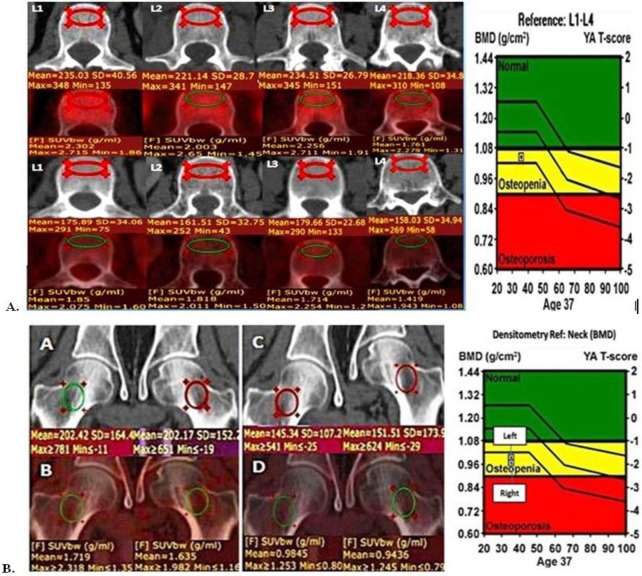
A. Initial staging (top rows) and post Chemo ± hormonal therapy (bottom rows) axial PET/CT images in a 37 year old female with IDC breast. Initial Staging PET/CT show normal L1 to L4 attenuation values (L1- L4 mean HU of 227) while post Chemo ± hormonal therapy scan revealed reduced lumbar vertebral attenuation (L1- L4 mean HU ~ 168 HU); an overall decline of 26%. Corresponding mean L1-L4 SUV_max_ values also demonstrated a decline from 2.58 to 2.04 ~ 20% decline. Post Chemo ± hormonal therapy DXA scan L1- L4 mean T- score was -1.3. B. Mean FNHU declined from 202.45 to 148.4 HU, a decline of ~ 54%, while mean L1-L4 SUV_max_ values also demonstrated a decline from 2.58 to 2.04 ~ 20% decline. Corresponding mean femoral neck T- score was -1.8
